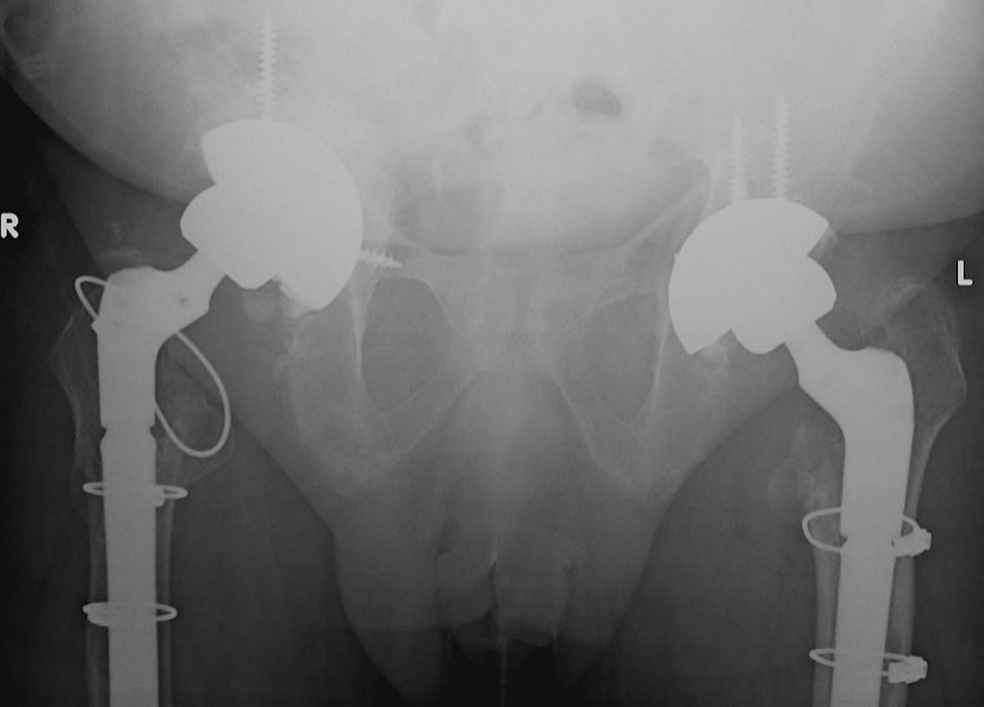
Final postoperative pelvis X-rays following left revision total hip arthroplasty, one year following revision on the right side
